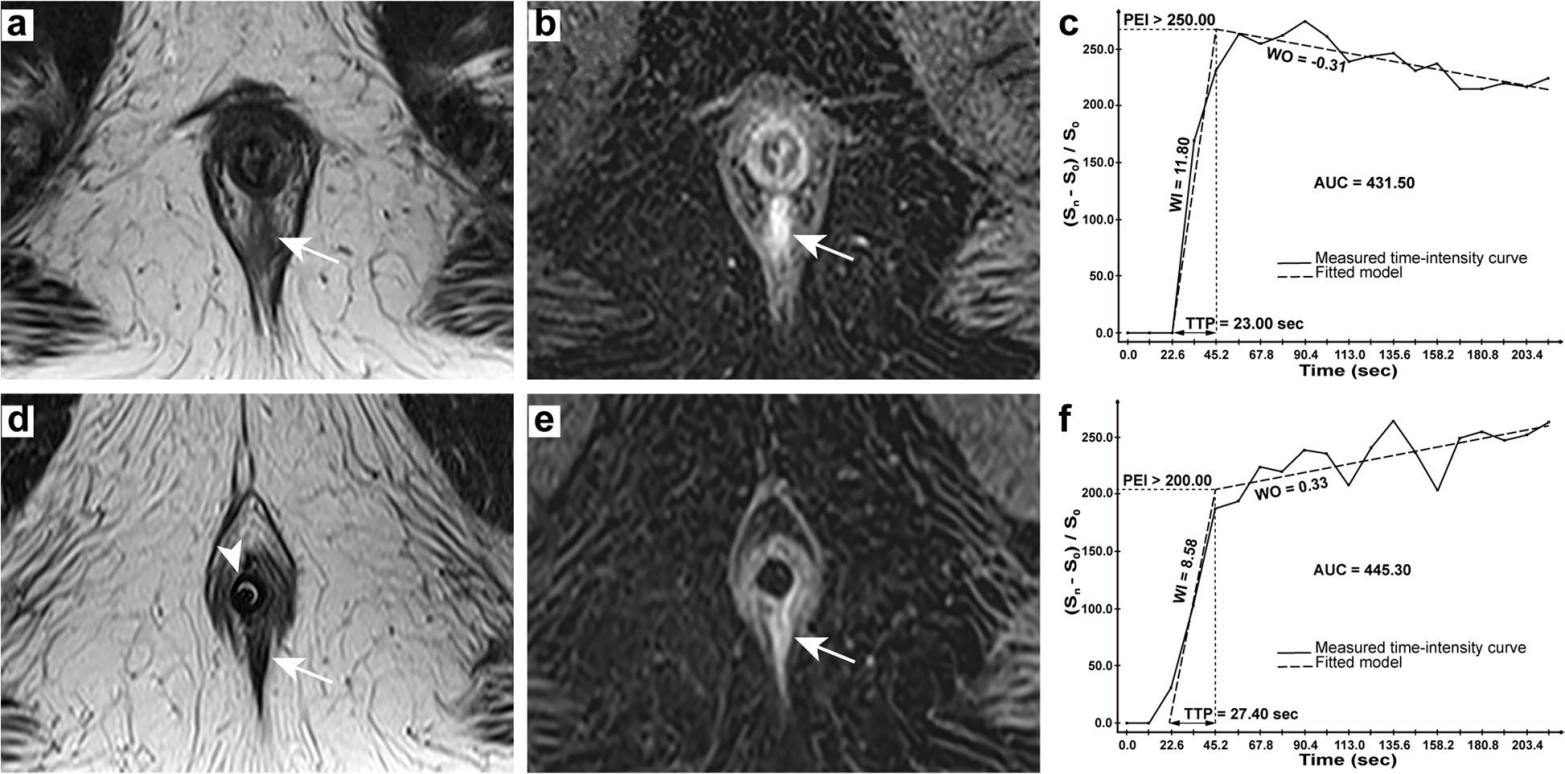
42-year-old man presenting a simple linear intersphincteric fistula. Preoperative MRI: axial T2-weighted image (a) showing the active fistula in hyperintensity (arrow), axial DCE image (b) showing early strong enhancement of the fistula (arrow) and TIC with parameters (c); Postoperative MRI: axial T2-weighted image (d) showing the healing tissue in hypointensity (arrow) and a balloon catheter (arrowhead), axial DCE image (e) showing progressive enhancement of the lesion (arrow) and TIC with parameters (f)
